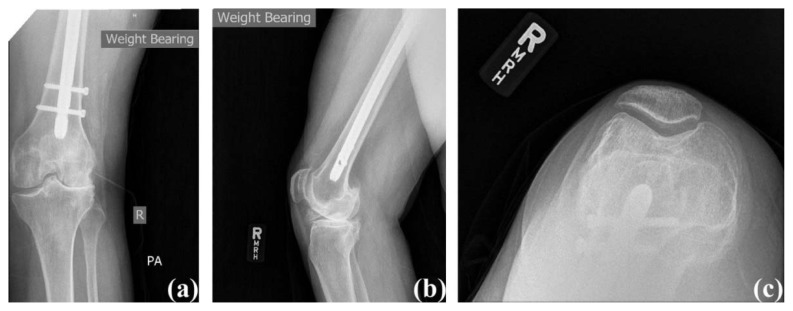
Case #1 pre-operative radiographs: (a) anterior posterior view, (b) lateral view, and (c) sunrise view.
